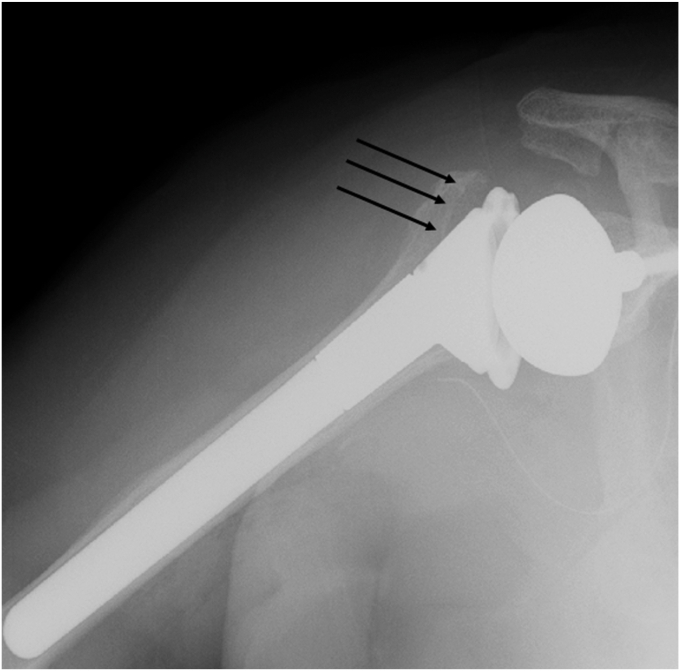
A radiolucent zone on the lateral side of the humerus on immediate postoperative radiographs. The medial portion of the stem did not fit into the medullary canal and was protruded.
